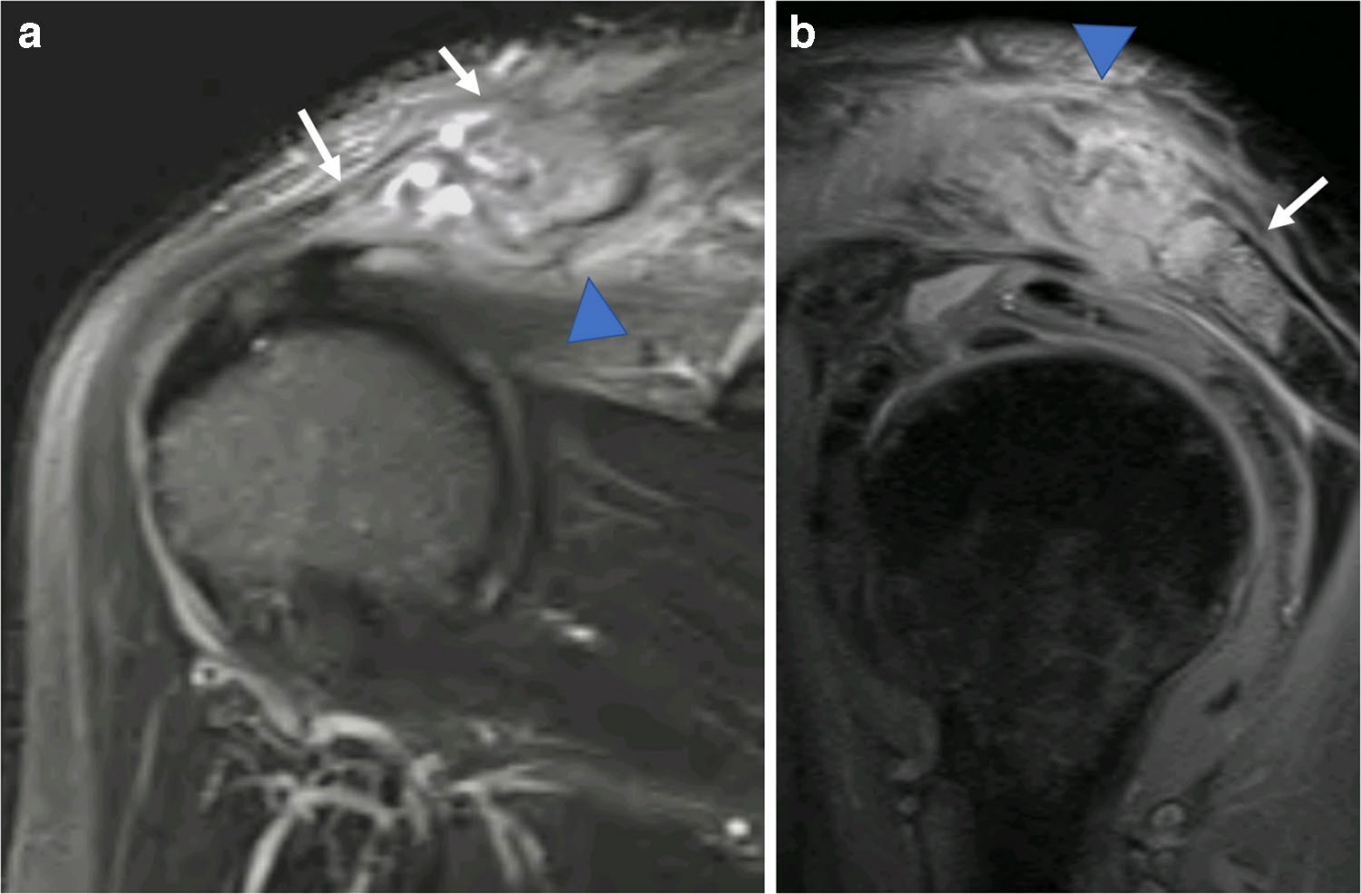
MRI of the left shoulder in a 65-year-old woman who presented with shoulder pain and fever 1 week after an ultrasound-guided acromioclavicular joint corticosteroid injection. Joint aspiration subsequently confirmed septic arthritis. Coronal (a) and sagittal (b) STIR images of the left shoulder demonstrate florid bone oedema spanning the acromioclavicular joint (white arrows), with marked periarticular soft tissue and subcutaneous oedema (arrowhead)
